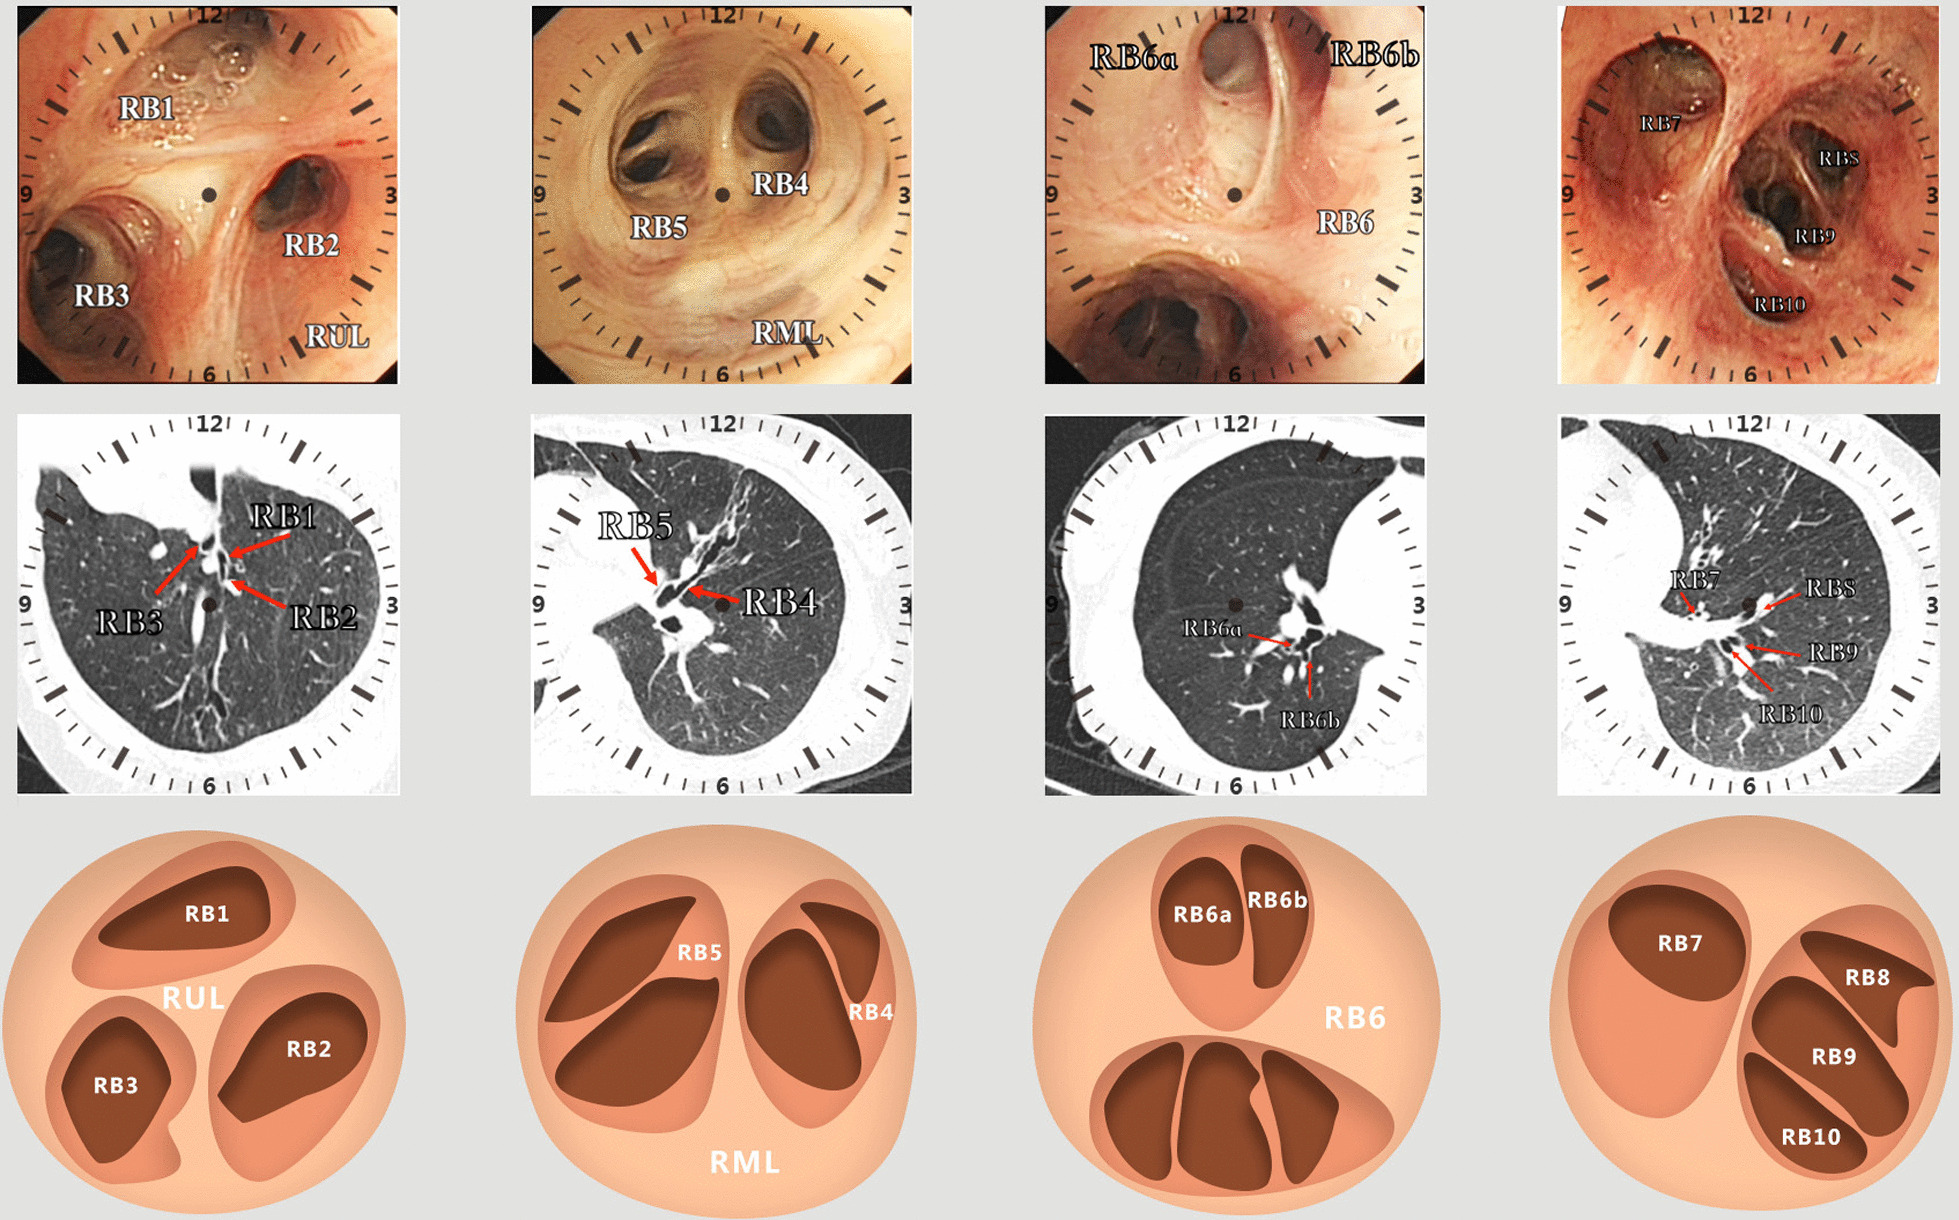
Bronchoscopy, CT image and navigation mapping of the left lung
